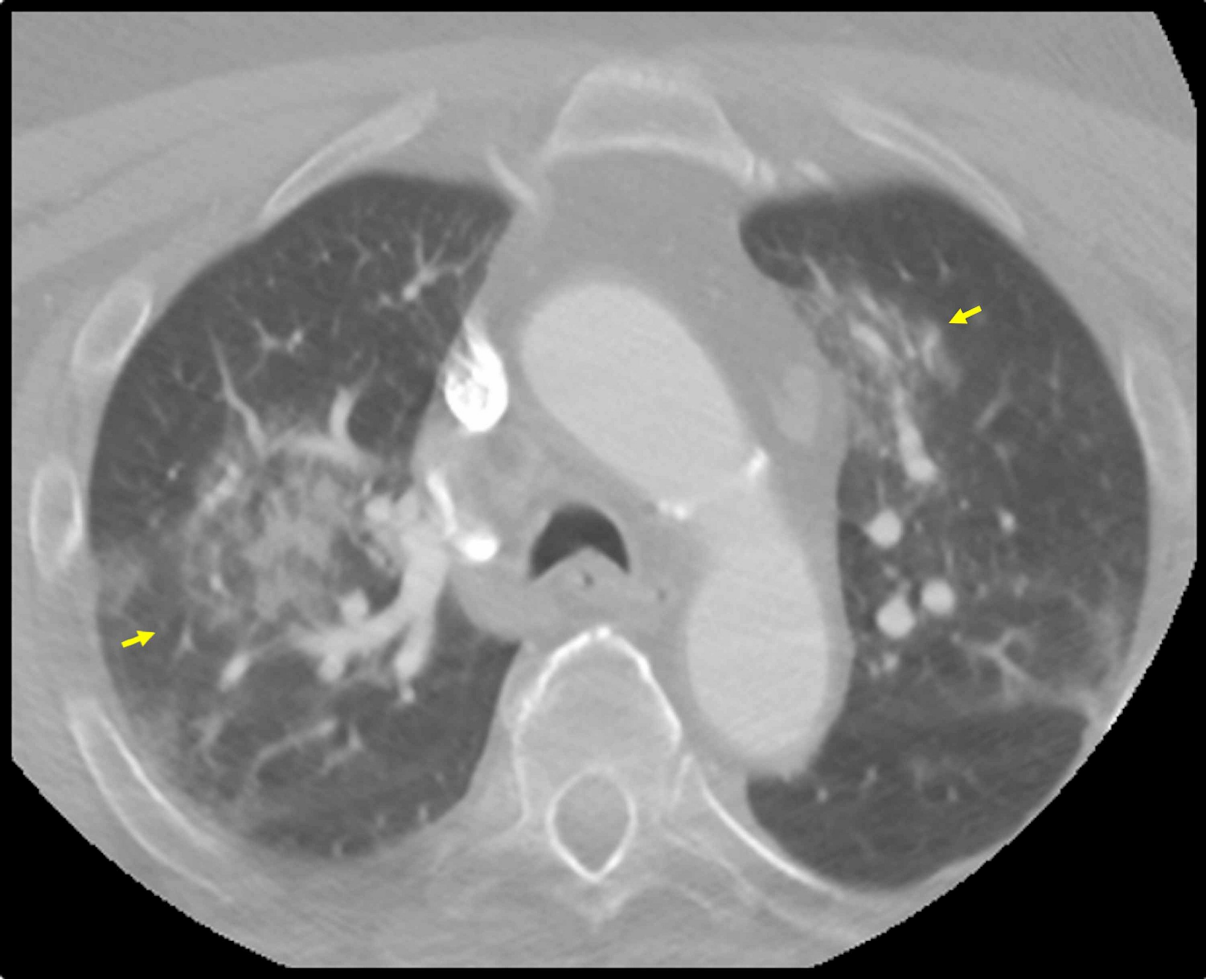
Axial image from a CT angiography of the head and neck at the level of the aortic arch The image displays randomly distributed ground-glass opacities bilaterally (yellow arrows) CT: computed tomography
